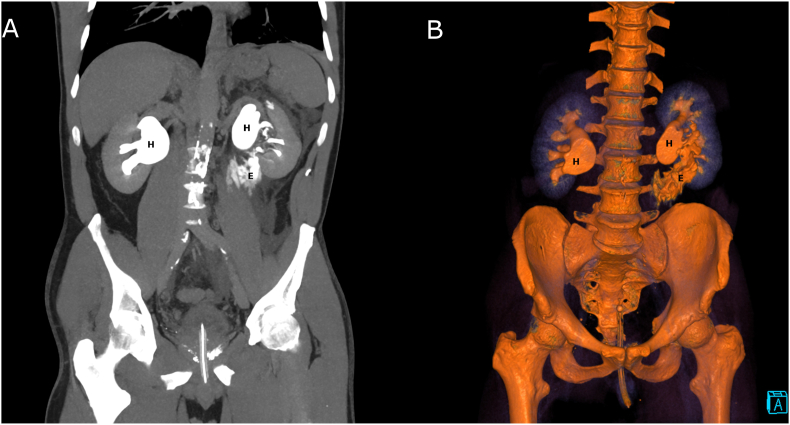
CT image; E, extravasation of the contrast from the ruptured ureter into the peri renal space; H, bilateral hydronephrosis. (A) CT coronal reconstruction. (B) CT 3D coronal reconstruction.
